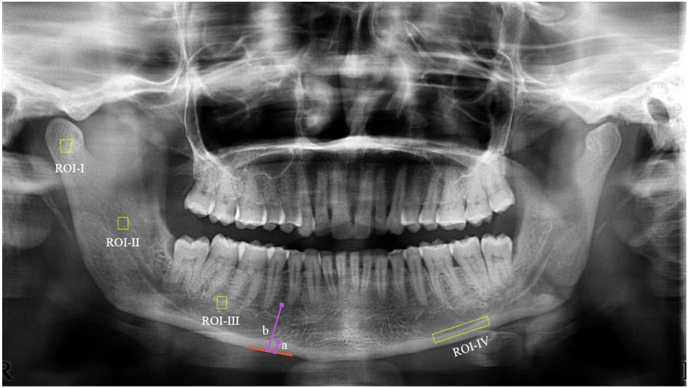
Selection of the region of interest. ROI-1 represents the central area of the condylar head without any degenerative conditions. ROI-2 corresponds to the central area of the ramus region. ROI-3 without periapical radiolucency or sclerosis below and between the molars. If a molar was absent, the middle area horizontally 2 cm medial from the oblique line-ramus intersection were chosen. ROI-4: The endosteal margin. Measurement of mental index line b measures mandibular cortical thickness at the mental foramen midway, perpendicular to the lower edge of the mandible. Measurement of Panoramic mandibular index line a measure mandibular cortical thickness at the mental foramen midway, perpendicular to the lower edge of the mandible.
